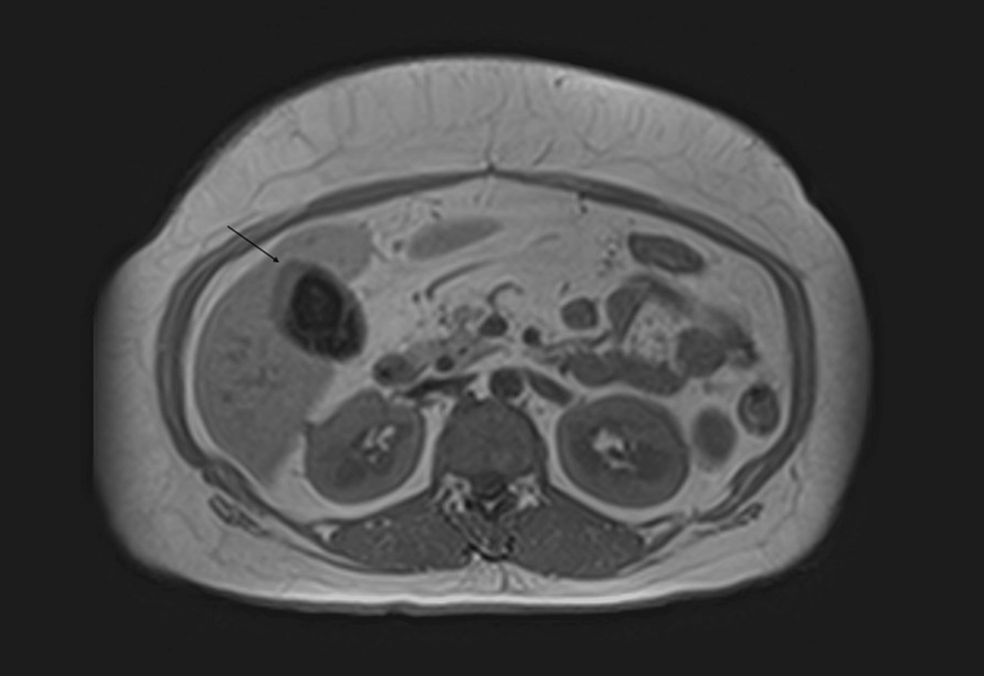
Abdominal MRI showing irregular wall thickening of the gallbladder fundus Wall thickening denoted by arrow
